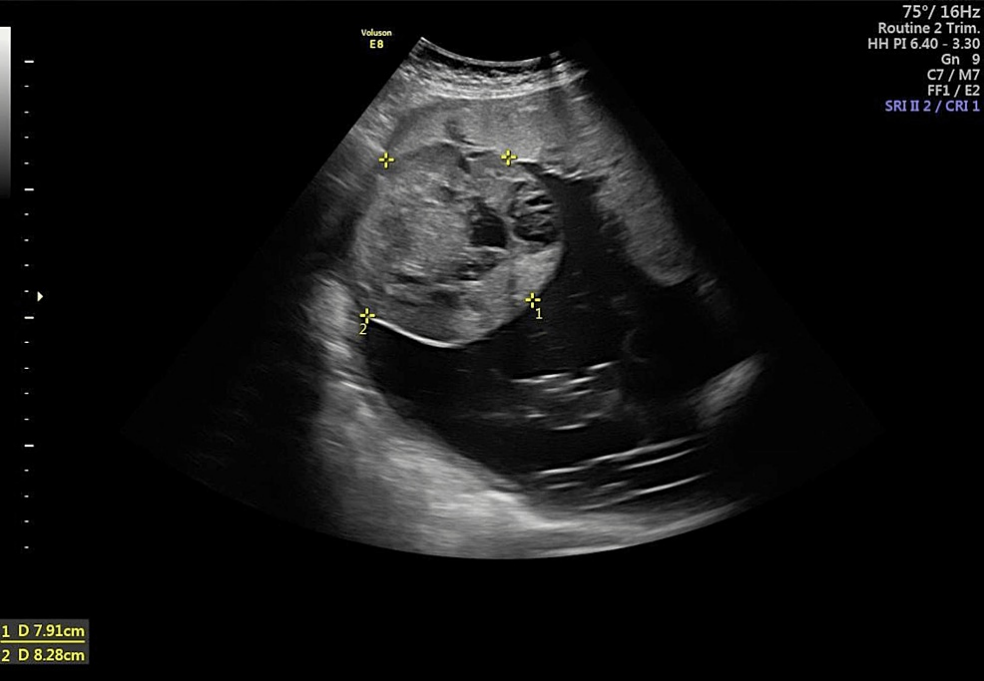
Ultrasound image at 31 weeks of gestation.
